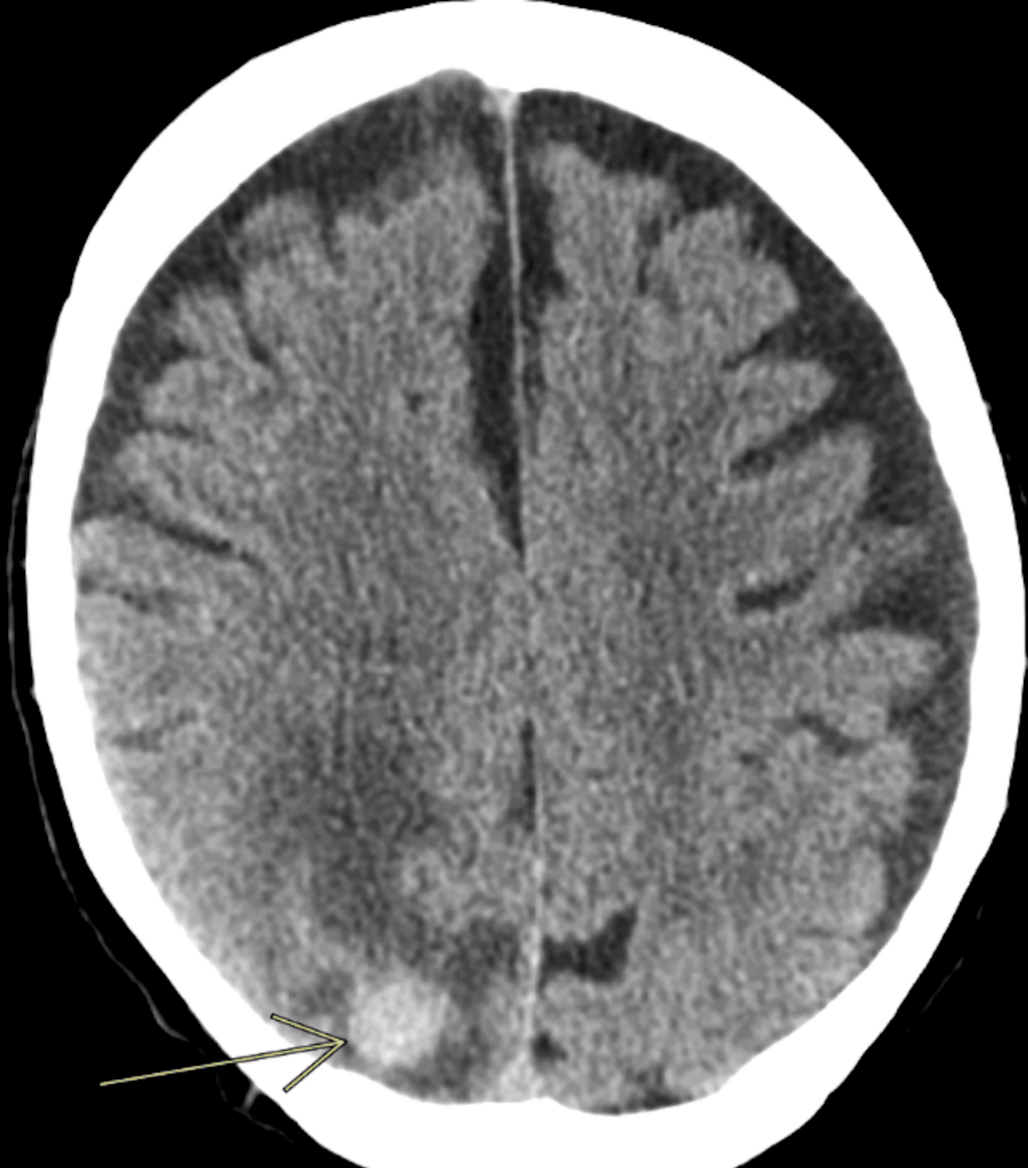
CT of the head/brain window revealing a rounded intra-axial 14 mm hyperdense right occipital lobe mass, presumed to be a metastasis from his Pancoast tumor with a moderate amount of perilesional white matter hypodensity/vasogenic edema The arrow points to the occipital lobe lesion
